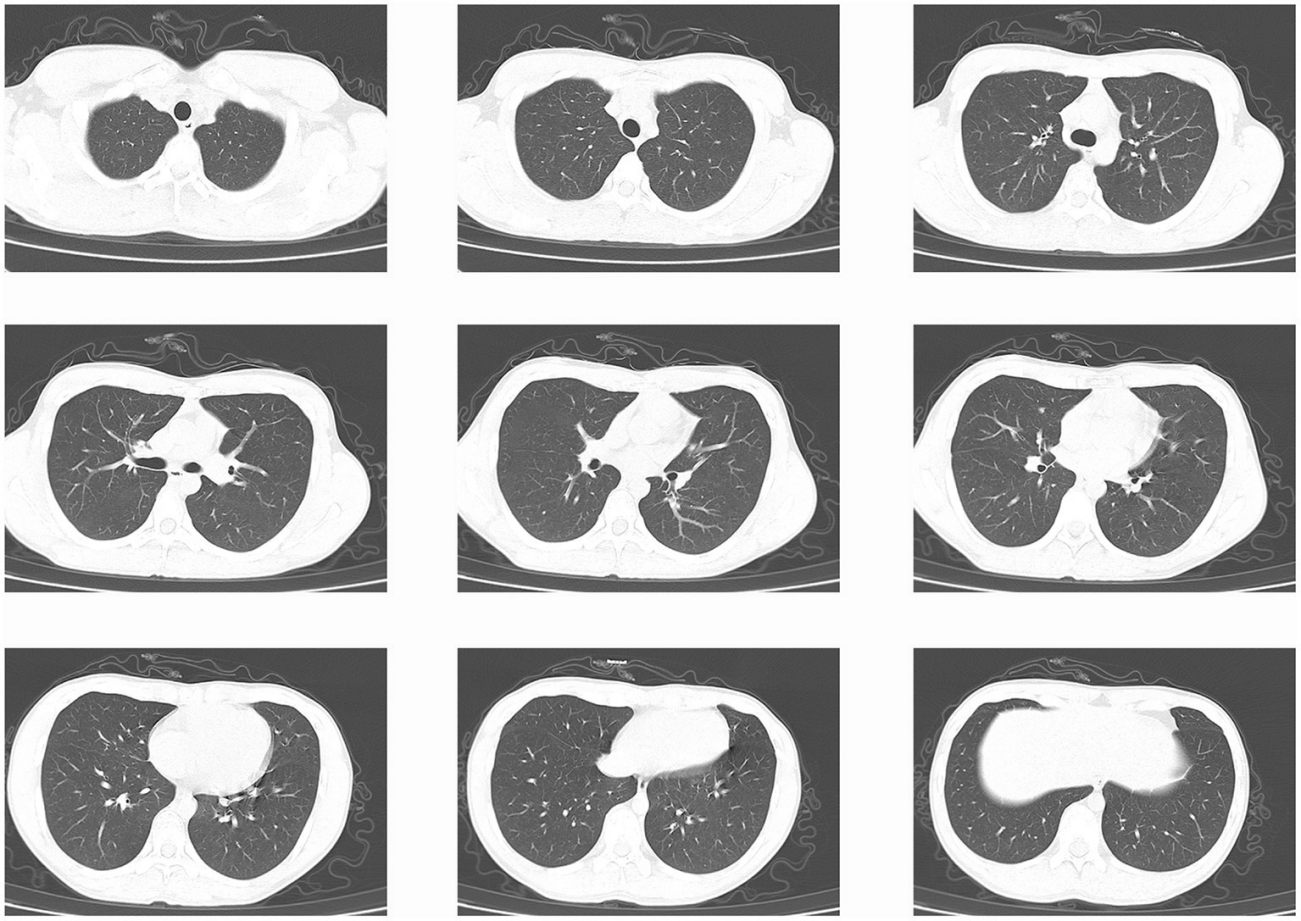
Chest computed tomography (CT) scan of the positive SARS-CoV-2 individual examined on March 13, 2020. The scans show healthy lungs based on the absence of abnormal shadow patch and the presence of ground glass shadow.
